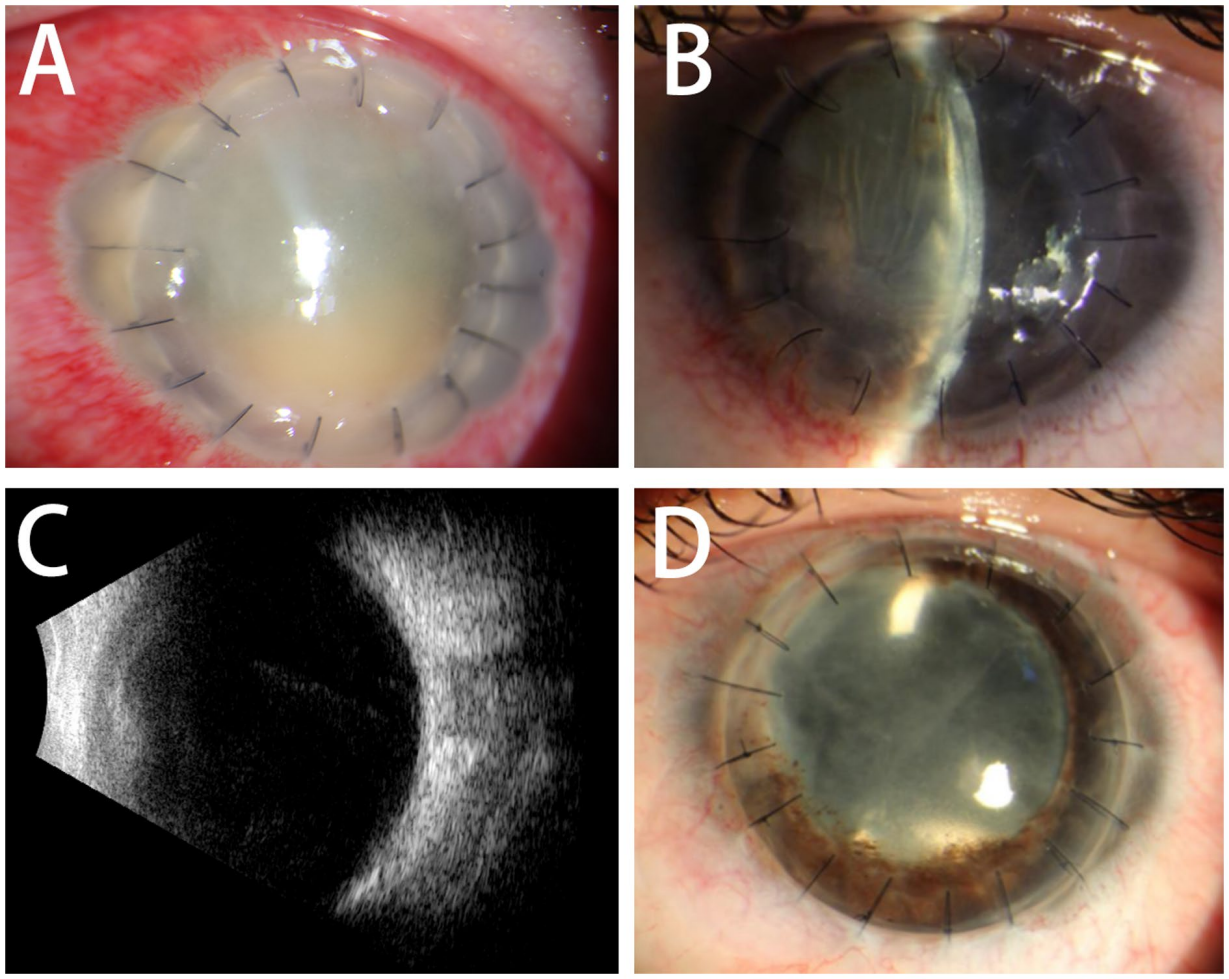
(A) The infection was infiltrated to the cornea and anterior chamber of the host. (B,C) The day after repeat-PKP, the graft is clear and the anterior segment is quiet with low intraocular pressure and lens opacity. The ultrasound showed vitreous opacity relief. (D) Six months later, the aided vision is 20/200 with a pin hole after cataract extraction and intraocular lens implantation.
